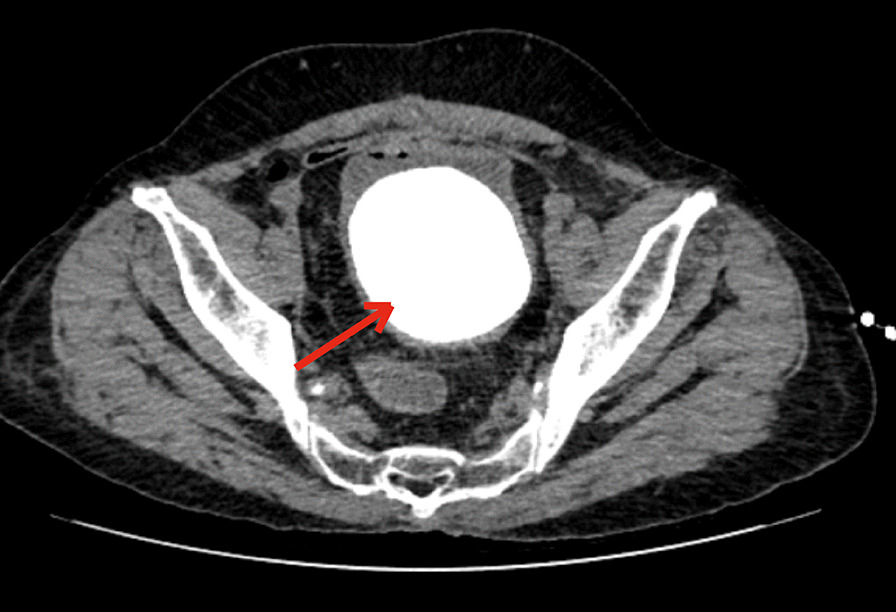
Nonenhanced pelvic CT scan. Notes: The red arrow shows a large stone (approximately 9.0 cm × 9.0 cm) in the neobladder
